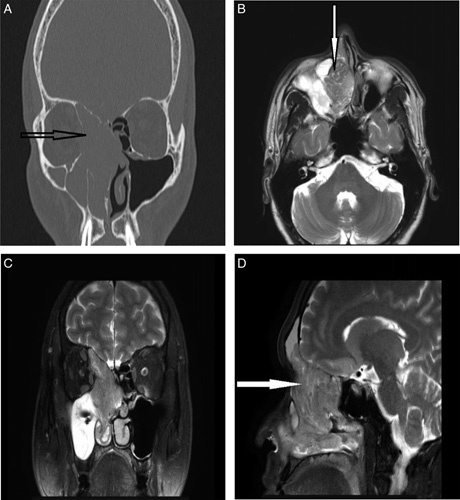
Coronal section image of CT PNS Bone window (A), Axial section image of MRI nose and PNS on T2W sequence (B), Coronal section image of MRI nose and PNS on T2W sequence (C), Sagittal section image of MRI nose and PNS on T2W sequence (D). PNS, paranasal sinuses.
